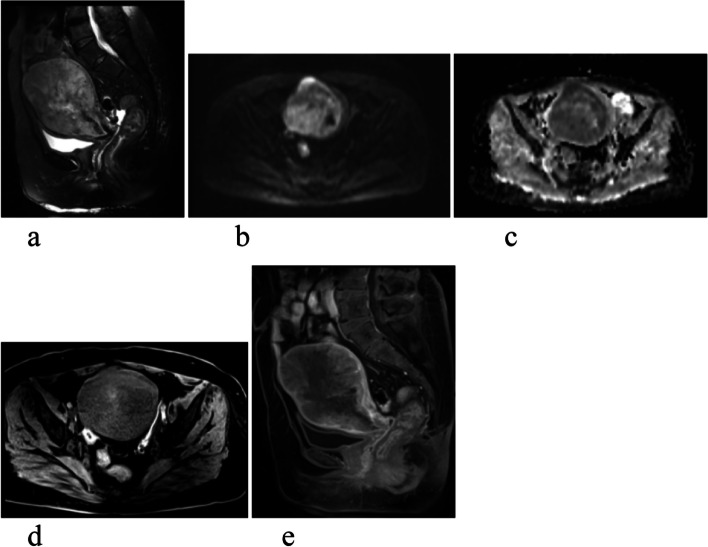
Images of uterine carcinosarcoma (UCS). a Sagittal T2WI of a patient shows a large intrauterine mass with heterogeneous high signal intensity. b Diffusion-weighted imaging (DWI) shows a high signal intensity tumor. c Apparent diffusion coefficient (ADC) displays mainly a low signal intensity tumor, some parts of high signal intensity. d Axial T1WI shows intratumoral hemorrhage of high signal intensity area in the mass. e Sagittal contrast enhanced T1WI shows a heterogeneous medium enhancement tumor, there is partial area without enhancement in the lesion
